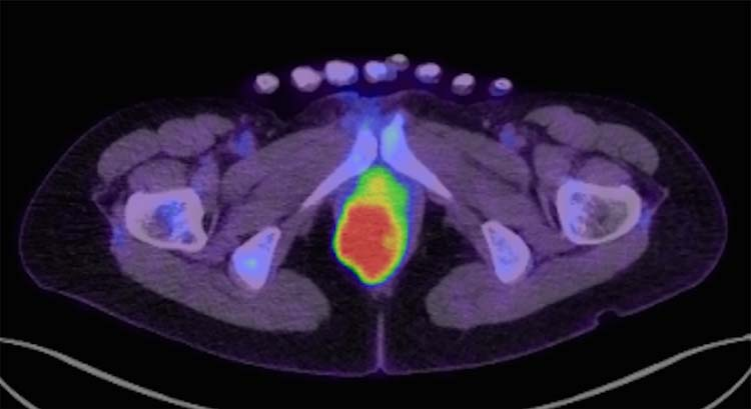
FDG-PET/CT (transverse section) show FDG accumulation in a mass with maximum standardized uptake of 17.9.
